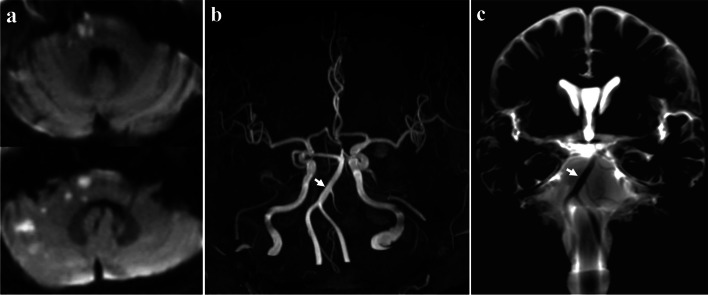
The diffusion-weighted image showed hyperintensity lesions in right inferolateral pons, brachium pontis and superior cerebellum (a). The right anterior inferior cerebellar artery (AICA) was absent on magnetic resonance angiography (b) and basi-parallel anatomic scanning magnetic resonance imaging (BPAS-MRI) (c)
